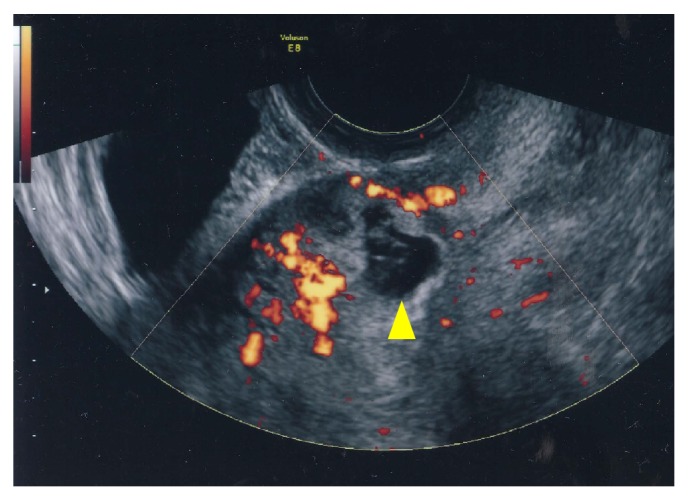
Transvaginal sonographic imaging. A midline sagittal view presenting the gestational sac at the uterine scar of a previous caesarean section and empty uterine cavity (power Doppler study). Triangle indicates the gestational sac.
